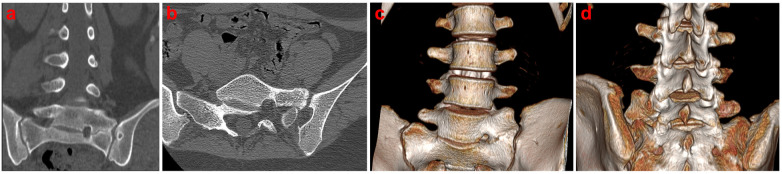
Preoperative sagittal (a) and axial (b) CT scans of the lumbar region, as well as the three-dimensional computed tomographic of the lumbar spine (c,d), revealed that the left transverse process of L5 was enlarged and formed an incomplete bony fusion with the sacrum through fibrous joint spaces.
